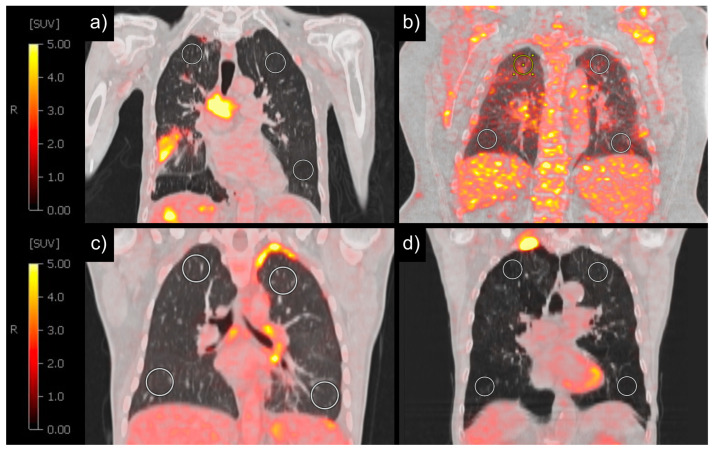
SUV measurements of the non-tumorous lung in four patients with a low BMI (15.3 kg/m^2^), resulting in a correspondingly reduced SUV_MEAN_ (0.25 g/mL) (a); with a high BMI (51.2 kg/m^2^), resulting in a correspondingly higher SUV_MEAN_ (0.99 g/mL) (b); without COPD (SUV_MEAN_ = 0.47 g/mL) (c); and with COPD (SUV_MEAN_ = 0.17 g/mL) (d). BMI = body mass index, SUV = standardized uptake value, COPD = chronic obstructive pulmonary disease.
